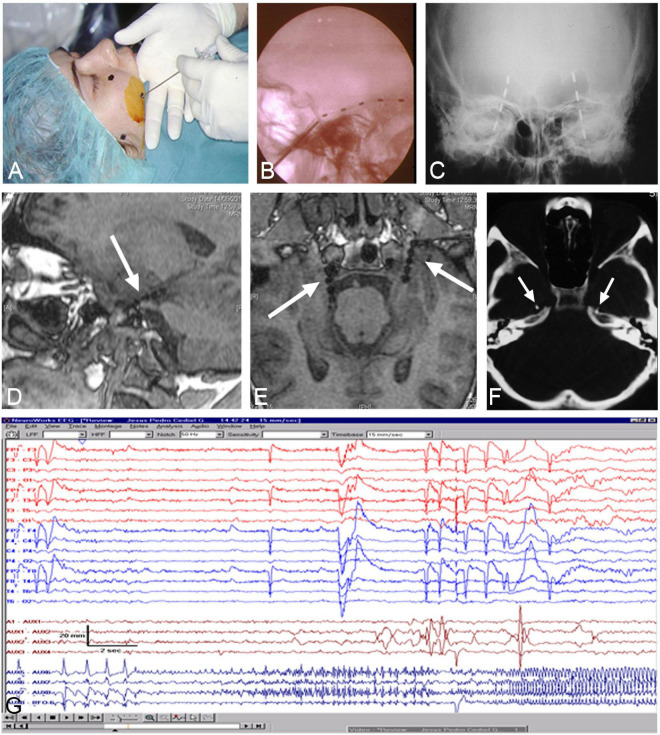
Foramen ovale electrodes (FOE). (A) Surgical image showing the insertion of the needle into the trigeminal (Gasser) ganglion cistern. (B,C) lateral and antero-posterior X-ray to assess the position of implanted FOE. (D–F) MRI and CT-scan to verify the position of FOE in the ambiens cistern, in contact with the uncus/parahippocampal gyrus (arrows). (G) Video-EEG recording revealing onset of seizures in contacts of the left FOE.
